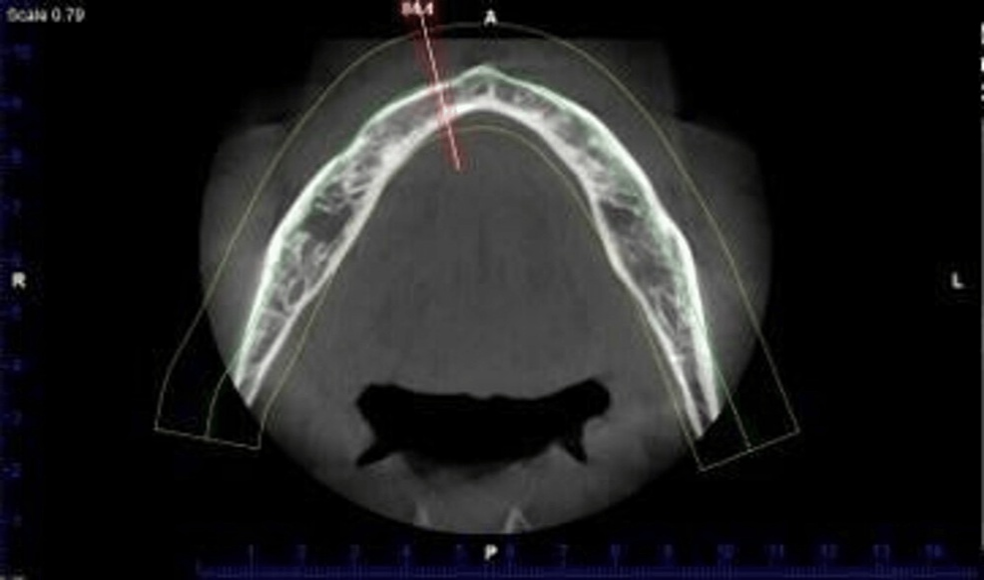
CBCT scans of the patients with PlanMeca Romexis software under ideal conditions of 84 kV and 14 mA. The stent was placed on the edentulous mandible and a CBCT scan was made. CBCT: cone beam computed tomography.
